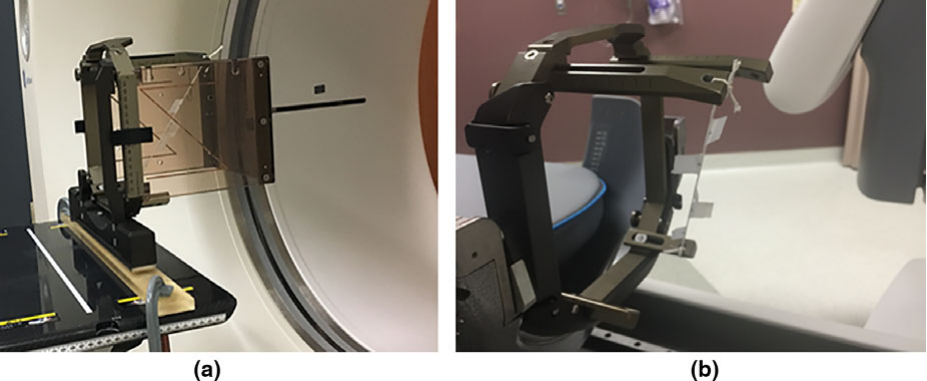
(a) CT scanning of the in‐house marker tool with the CT indicator box attached to; and (b) the same tool mounted on the Gamma Knife Icon couch adapter in preparation for CBCT.
